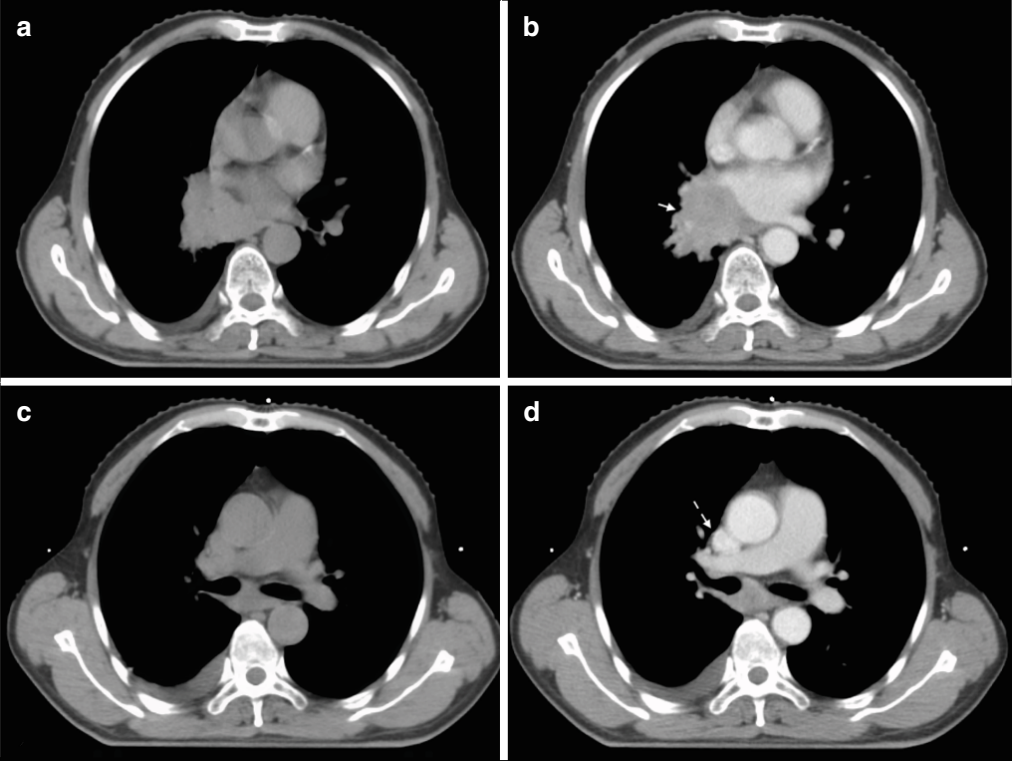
The Hounsfield units (HUs) of primary lesion in plain 3D-CT (a) and CE-4D-CT (b) were 56 ± 7 and 71 ± 10, respectively; the HUs of mediastinal lymph nodes in plain 3D-CT (c) and CE-4D-CT (d) were 39 ± 6 and 55 ± 15, respectively. The lesion in a and the lymph node in c are ill-defined, but well-defined in enhanced b (*solid arrow*) and d (*dotted arrow*)
